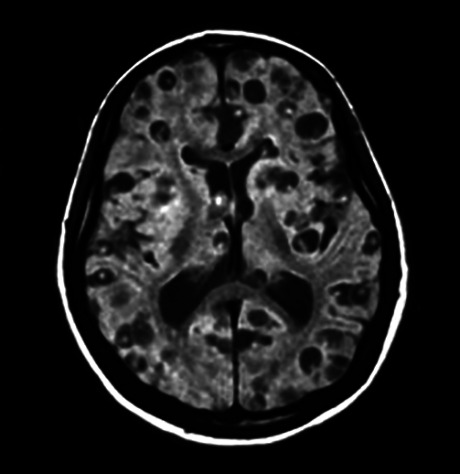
MRI multiple intraparenchymal viable cysticerci.
